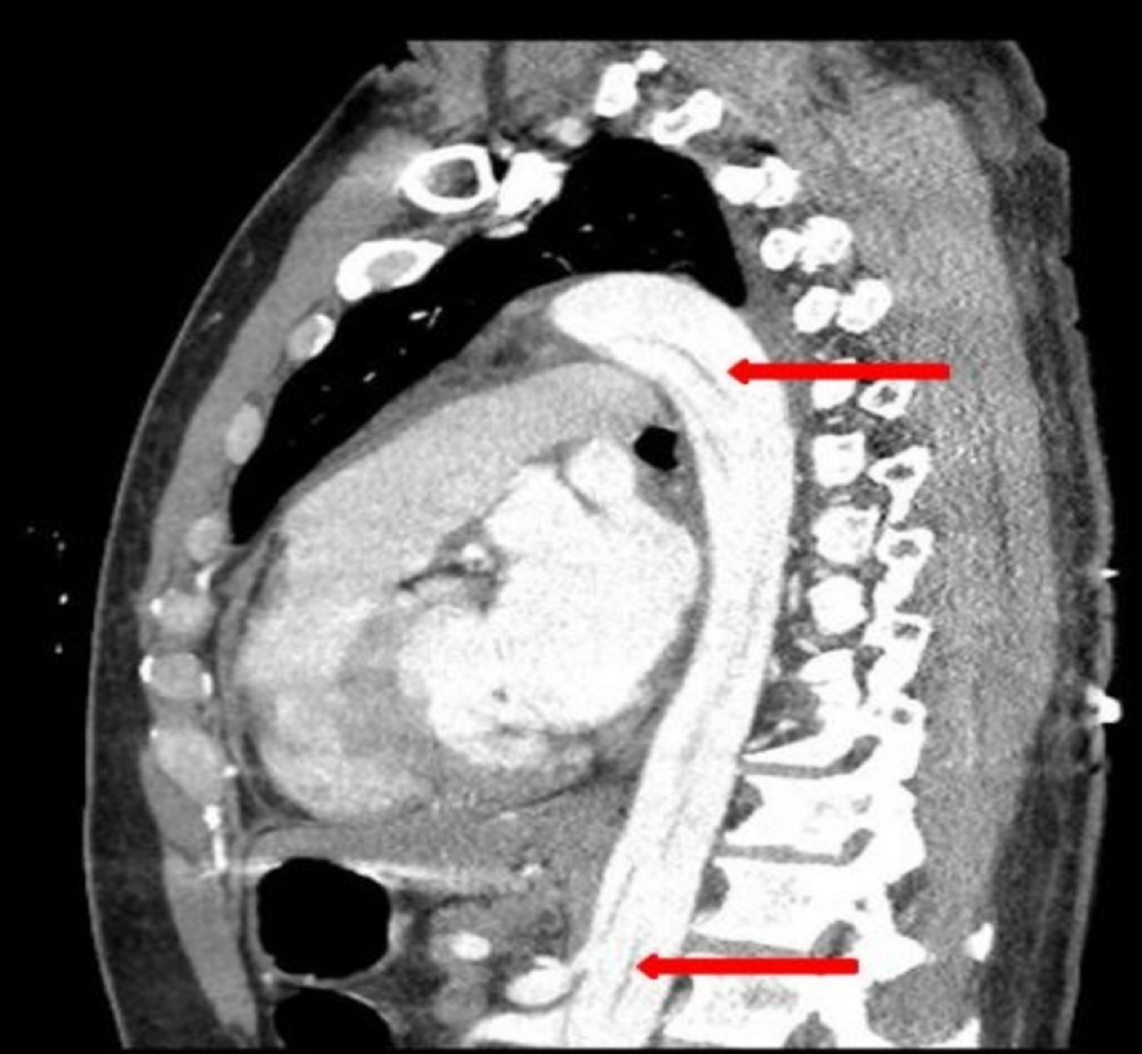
Computed tomography scan showing evidence of dissection in the aortic arch (upper arrow) and extension into the abdominal aorta (lower arrow).
